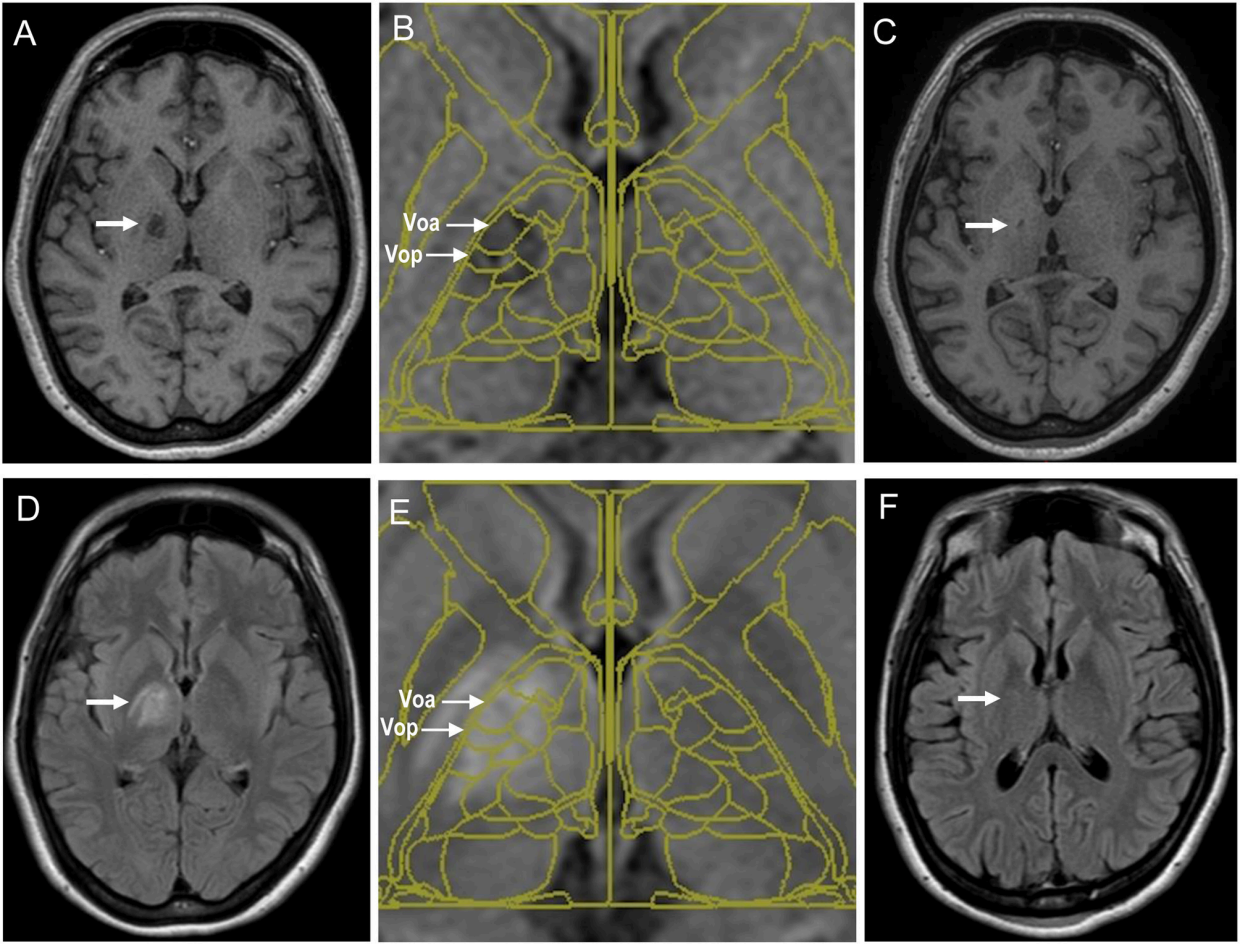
Magnetic resonance images of coagulative lesions located in the thalamic Vo nucleus of a patient with writer's cramp who underwent stereotaxy. (A–C) The T1-weighted acquisition images at 4 days (A) and 1 year (C) after the surgery are shown. Fusion of the Schaltenbrand and Wahren atlas with the T1-weighted acquisition image at 4 days after the surgery is also shown in (B). Coagulative lesions are indicated by arrows (D–F). The fluid-attenuated inversion recovery (FLAIR) images at 4 days (D) and 1 year (F) after the surgery are shown. Fusion of the Schaltenbrand and Wahren atlas with the FLAIR image at 4 days after the surgery is also shown in (E). Coagulative lesions are indicated by arrows. Voa, the ventralis oralis anterior nucleus; Vop, the ventralis oralis posterior nucleus; Vim, the ventralis intermedius nucleus; Vc, the ventralis caudalis nucleus.
